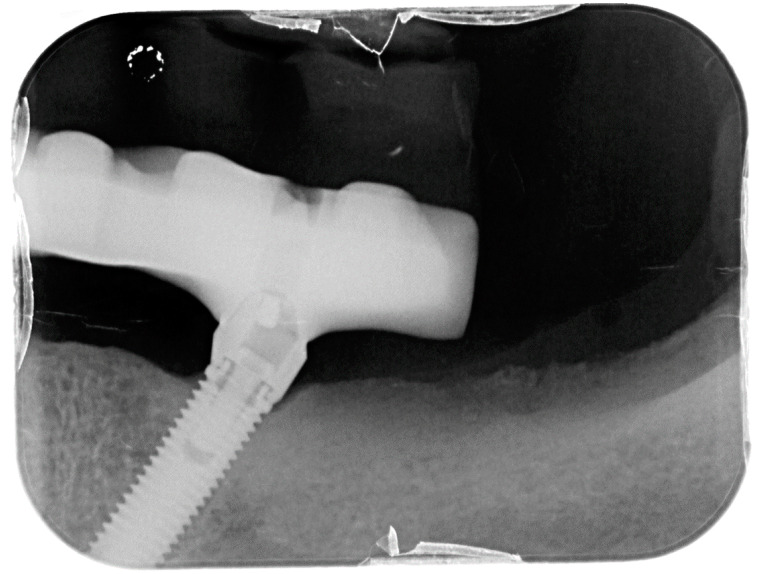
X-ray of 3.5 dental implant in patient in 6 months after treatment.
